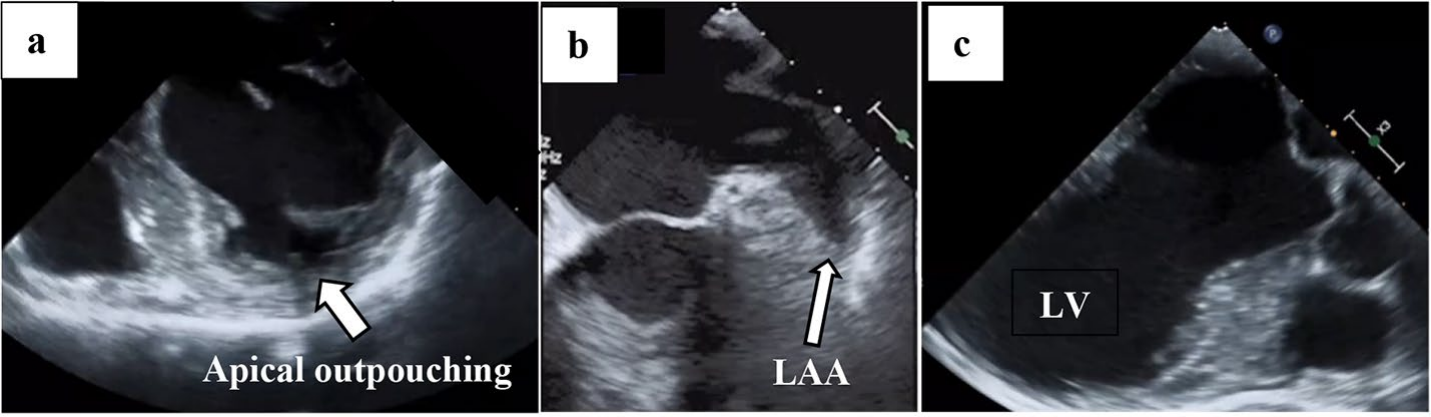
Intraoperative transesophageal echocardiography. a Apical outpouching of the left ventricle. b Left atrial appendage. c Left ventricle. LV: Left ventricle, LAA: Left atrial appendage.
